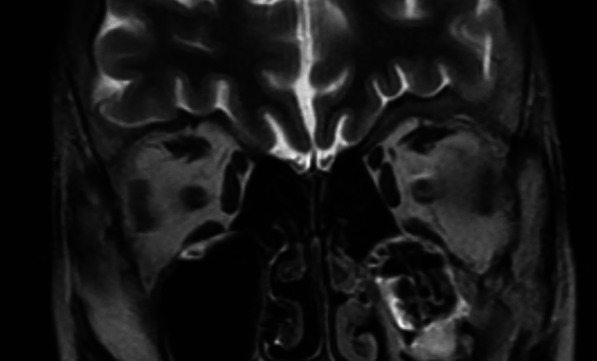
Hypothalamic–pituitary MRI showing hypoplasia of the olfactory bulbs
